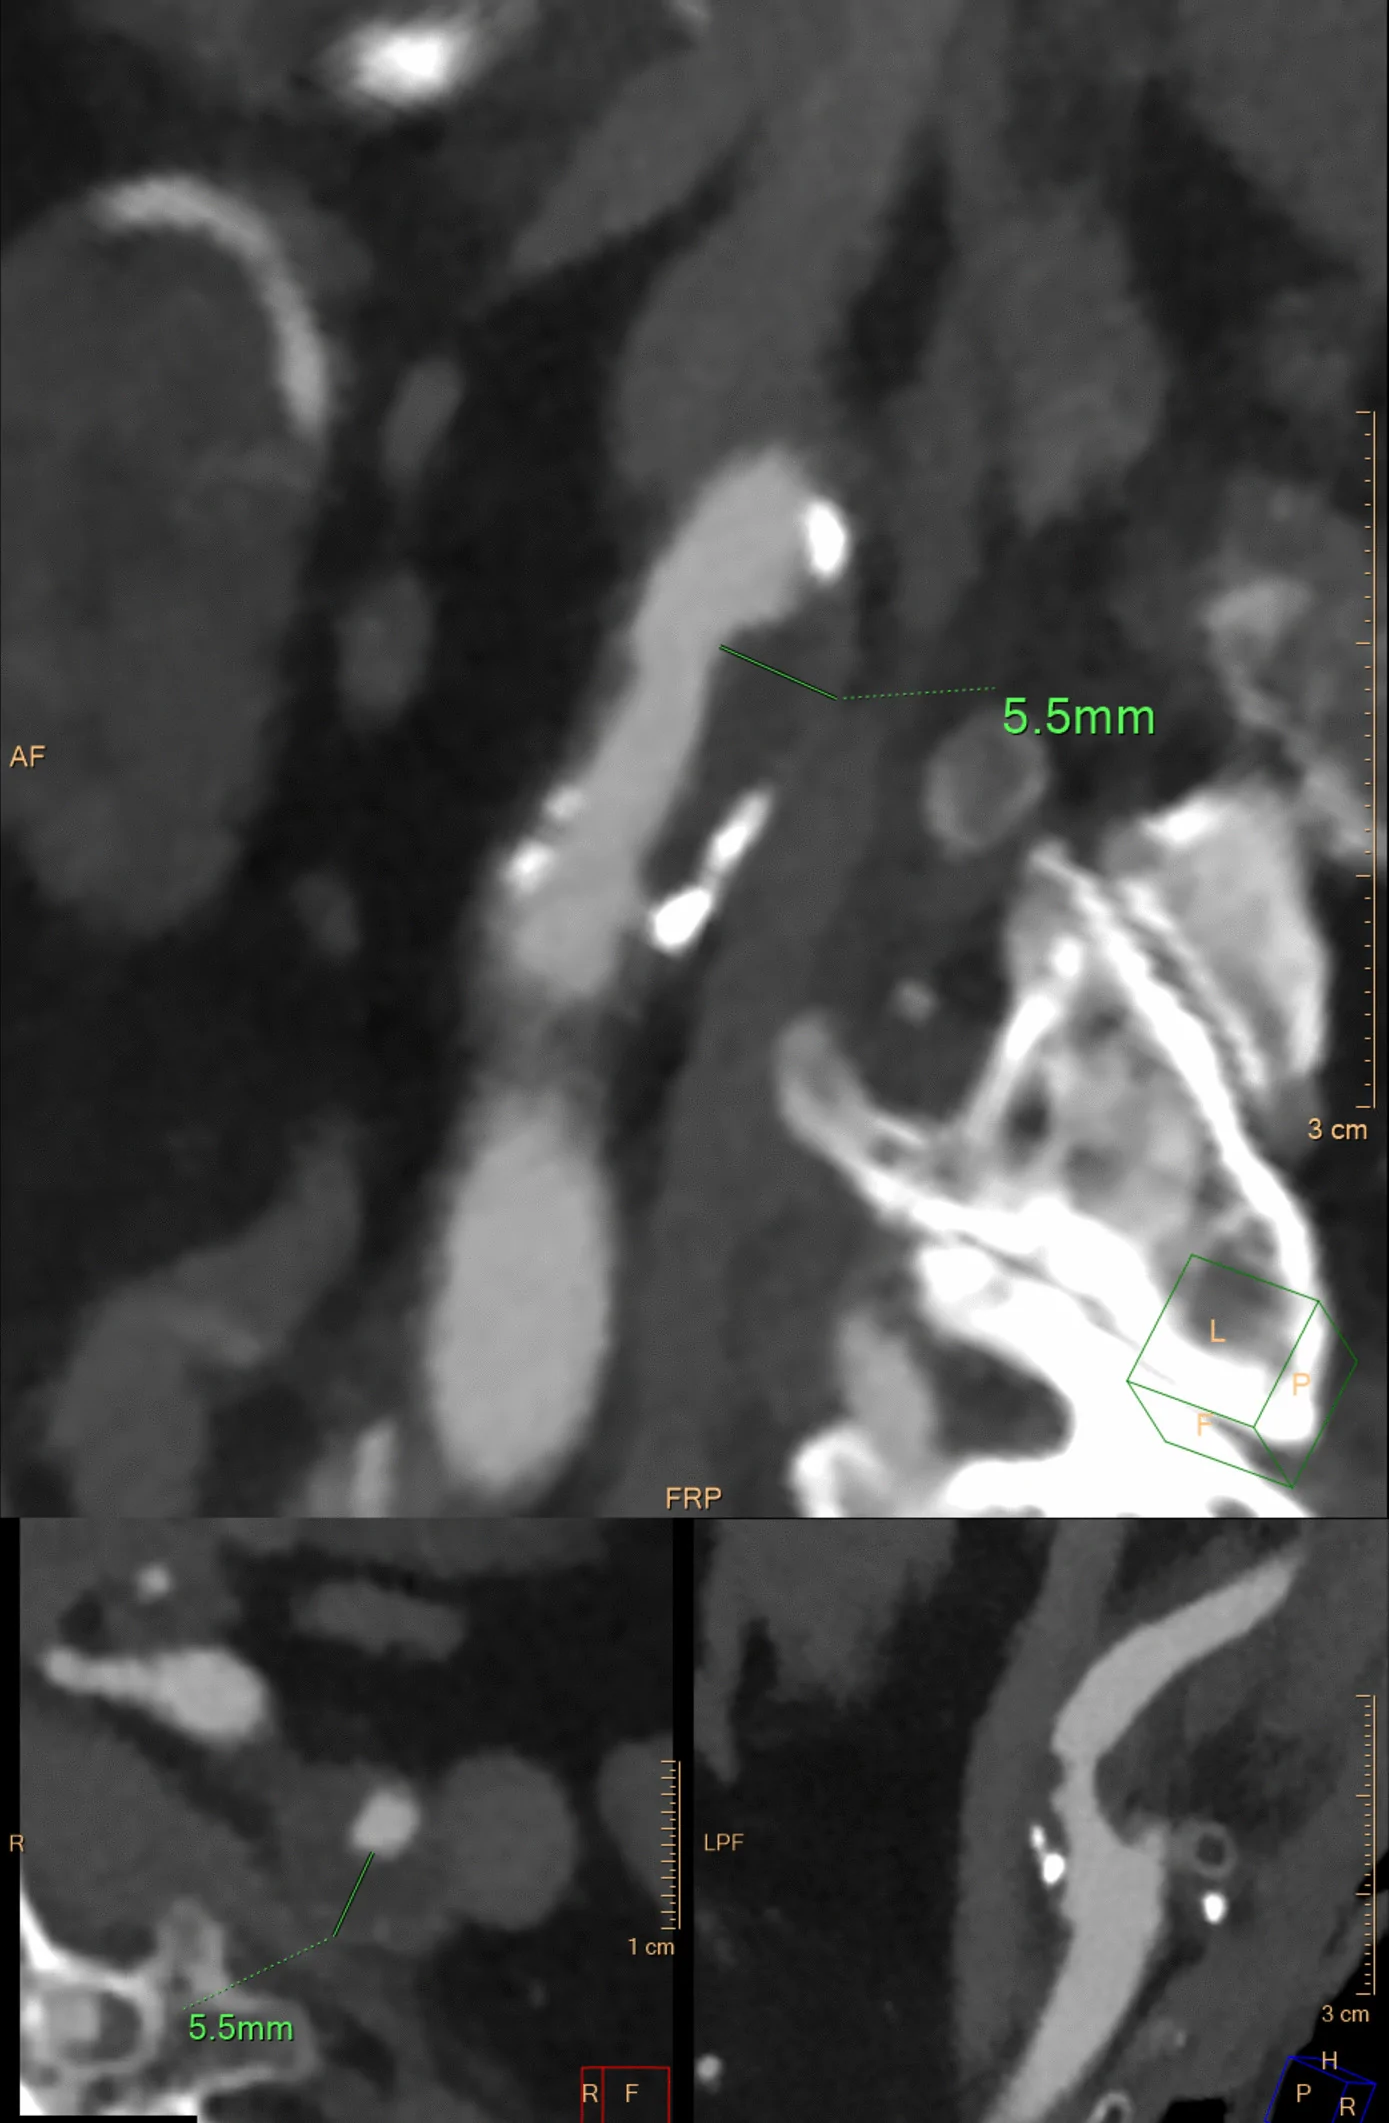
Multiplanar CT reformats perpendicular to the residual lumen of the stenosed right internal carotid artery, with measurement at the site of maximal plaque thickness (*green text*)
